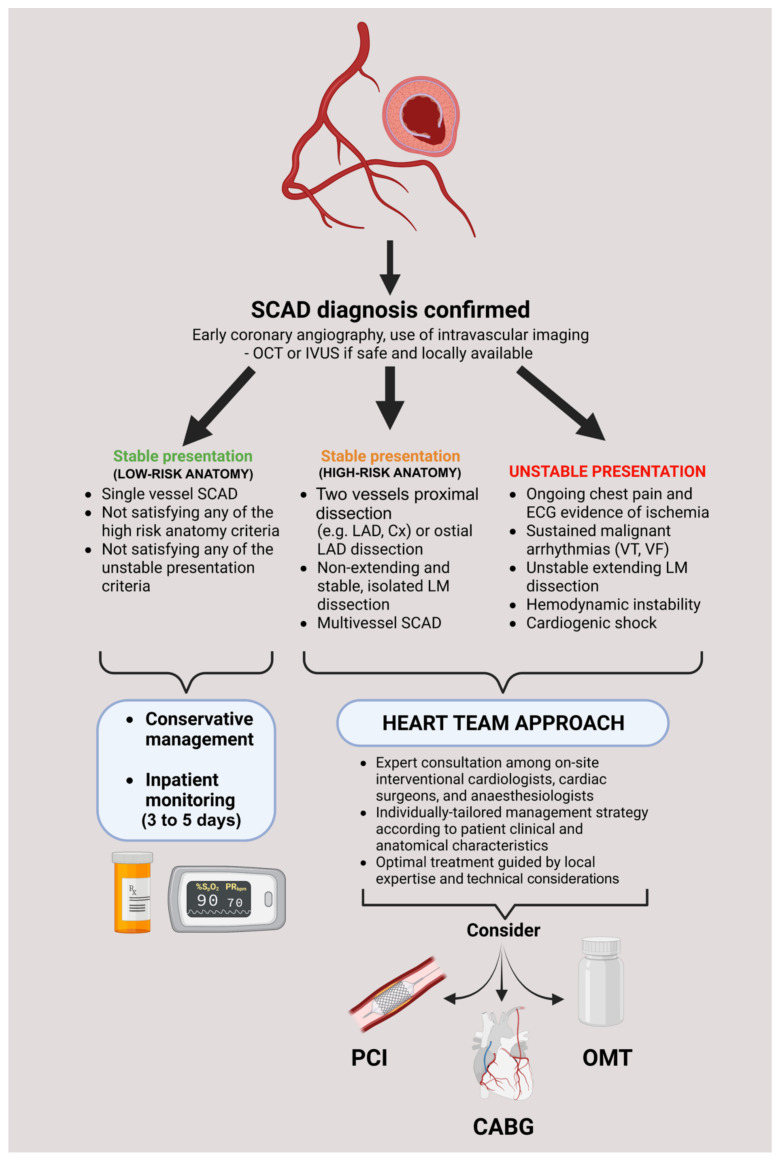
A decision tree in approaching confirmed SCAD cases during coronary angiography. Abbreviations: CABG: coronary artery bypass grafting; Cx: circumflex artery; IVUS: intravascular ultrasound; LAD: left anterior descending artery; LM: left main; OCT: optical coherence tomography; OMT: optimal medical therapy; PCI: percutaneous coronary intervention; VF: ventricular fibrillation; VT: ventricular tachycardia.
